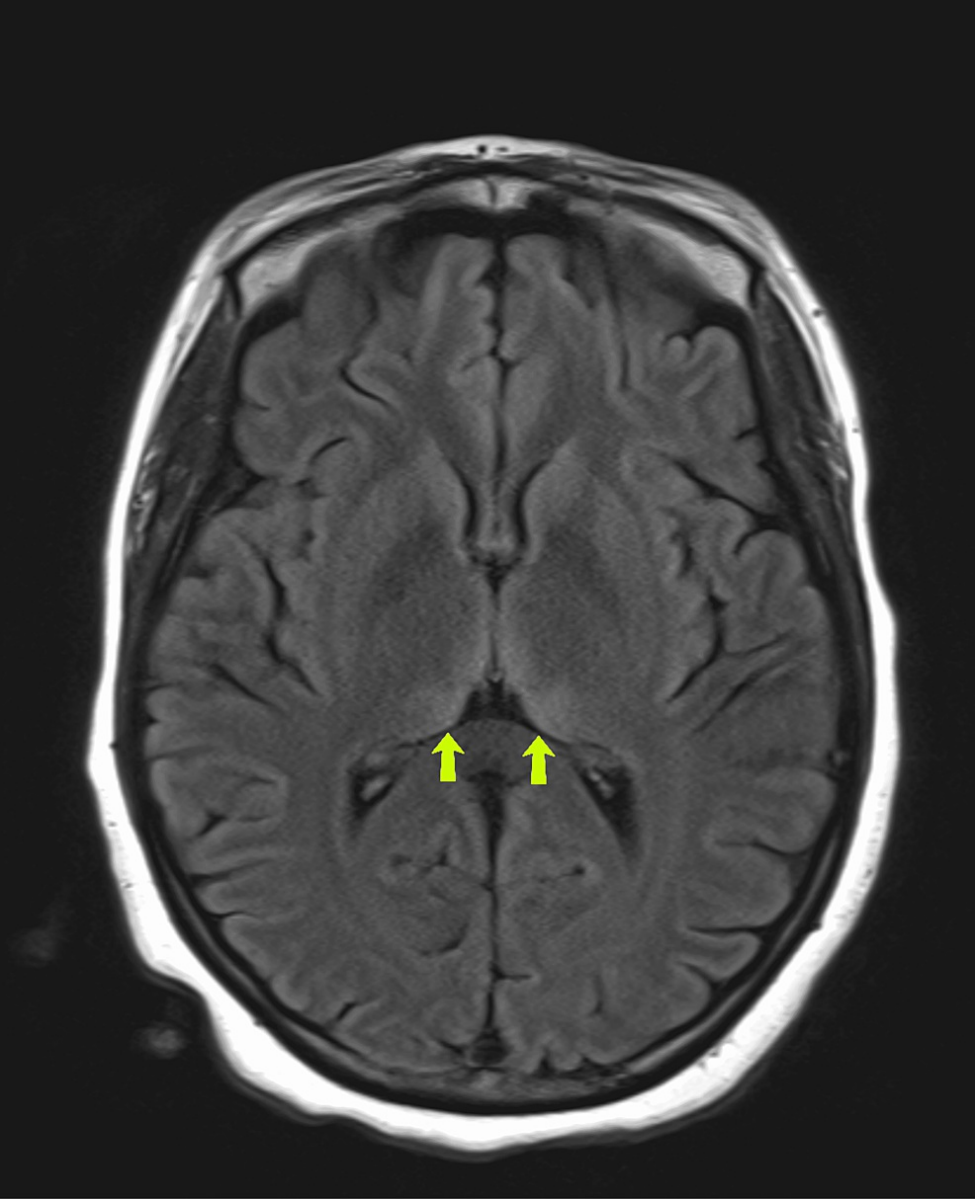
Magnetic resonance imaging (MRI) brain with and without contrast showing increased T2 signal in the medial thalamus bilaterally (yellow arrows), consistent with Wernicke’s encephalopathy performed during hospital admission.
